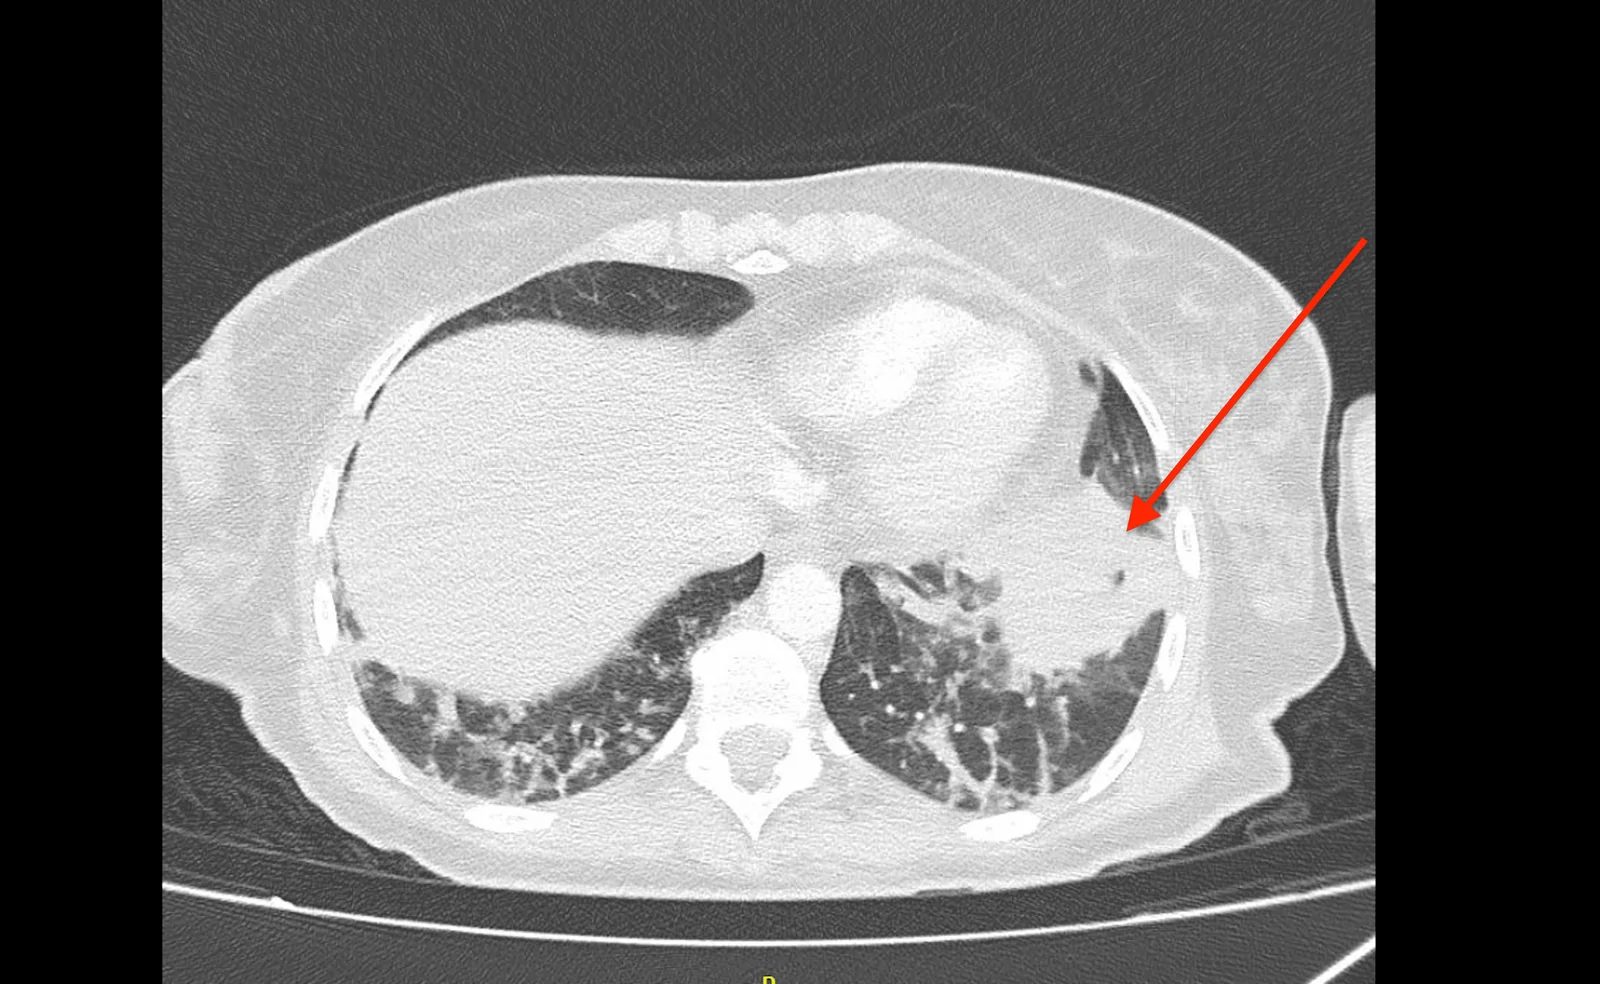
Contrast-enhanced computed tomography (CT) thorax demonstrating septic pulmonary embolisation Axial CT image of the thorax showing peripheral nodular air-space consolidation within the left lower lobe (arrow), consistent with septic pulmonary embolisation. Additional bilateral patchy bronchopneumonic infiltrates were also present.
